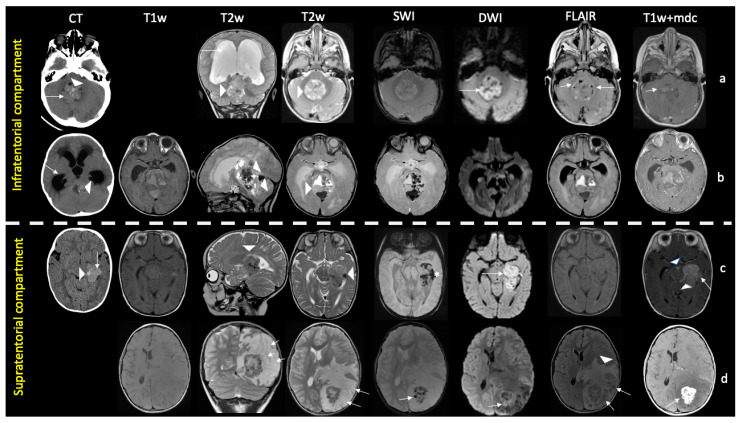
Imaging findings of pediatric ATRT in supra- and infratentorial compartments. Infratentorial ATRTs (rows (a,b)). Row (a): One-year-old child with a prevalently solid mass centered in the IV ventricle, hyperintense to gray matter (arrowheads in T2w) with high density/restricted diffusion (arrows in CT, DWI) due to hypercellularity, minute eccentric cystic components (arrows in FLAIR), and mild enhancement (arrow in T1+mdc). Intratumoral foci of Ca++ (arrowhead in CT) are present, interstitial edema is absent. Hydrocephalus is shown (white arrow in T2). Row *(*b): a 4-month-old child with a bulky heterogeneous mix mass with epicenter in the posterior cranial fossa (IV ventricle and vermis) and supratentorial extension (arrowheads in T2w). The lesion contains small hyperdense foci of Ca++ (arrowhead in CT), hemorrhage both in solid and cystic component (hyperintense signal in T1w, hyperintense and hypointense signal in T2w, hypointense signal in SWI). Interstitial edema is absent. Hydrocephalus is detected (white arrow in CT). Supratentorial ATRTs (rows (c,d)). Row (c): a 7-month-old child with a solid and heterogeneous intracranial mass centered in the temporal lobe, near to lateral ventricle (arrowheads in T2w). It shows high density (arrow in CT) and restricted diffusivity (arrow in DWI) due to hypercellularity, containing foci of Ca++ (arrowhead in CT), areas of hemorrhage (* in SWI), and marked enhancement (arrow in T1+mdc). Note widespread subarachnoid metastatic disease filling the interpeduncular and perimesencephalic cisterns (arrowheads in T1+mdc). Row *(*d): a 10-year-old child with a mixed lesion in the parietal lobe consisting of a heterogeneous solid component due to hypercellular (arrow in DWI) and hemorrhagic areas (arrow in SWI) and multiple eccentric cysts (arrows in T2 and FLAIR); note marked enhancement (arrow in T1+mdc) of the solid component and abundant perimarginal edema (arrowhead in FLAIR).
